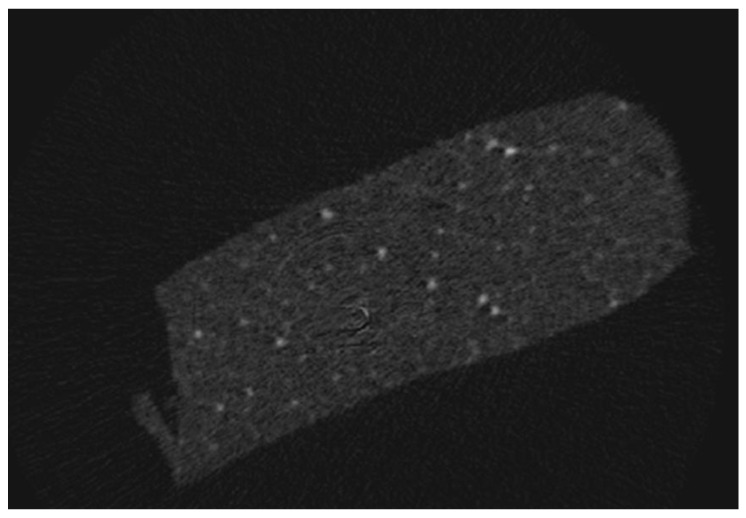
X-ray SKYSCAN 1174 microtomograph (8% ceramic nanofiller in epoxy matrix).
